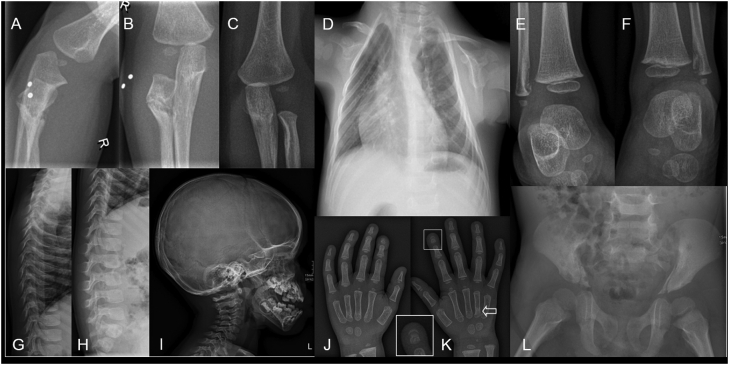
Radiographs made as part of the diagnostic work-up at presentation. A, B, C. Anteroposterior (AP) and lateral radiograph of the right (two radiopaque markers) and AP left elbow. The radiograph of the right elbow was initially interpreted as a fracture of the proximal radius. The radius shows a metaphyseal well-demarcated lucency with local angulation. The AP radiographs shows sclerotic margins of the lesion. The lateral side of the metaphysis of the left radius shows a cortical irregularity and slight bowing. D. Slightly oblique AP chest radiograph shows the deformity of the chest with slender down slanted ribs. E, F. AP radiographs of the ankles shows symmetrical metaphyseal cortical irregularity of the medial side of the fibulas. G, H, I. Normal development of the thoracolumbar spine and skull. J, K. Posteroanterior (PA) radiograph of both hands, there is some sclerosis at the base of the fourth metacarpal of the right hand (arrow). The tuft of the second digit of the right hand is irregular (insert). L. AP pelvis, note the linear irregularities on the inside of the iliac bones. There is slight flaring of the iliac bones and mild dysplasia of the hips.
